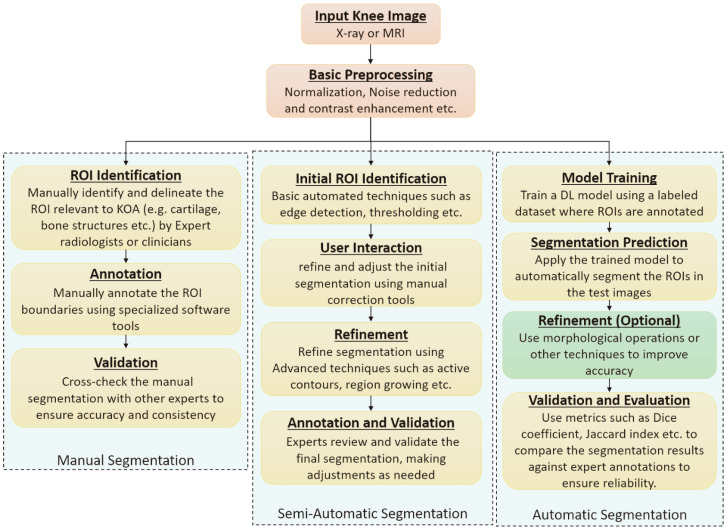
Overview of manual, semi-automatic, and automatic segmentation techniques for knee radiographs.
